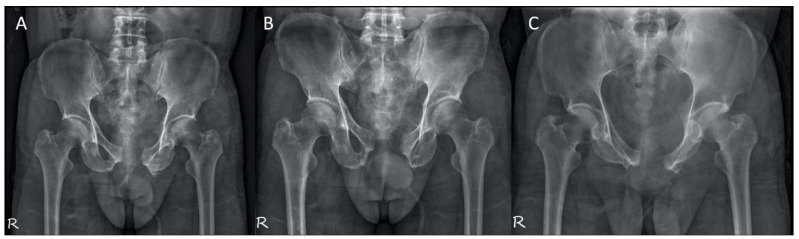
Preoperative X-ray films of the pelvis. (A) AP view, (B) outlet view, and (C) inlet view.
